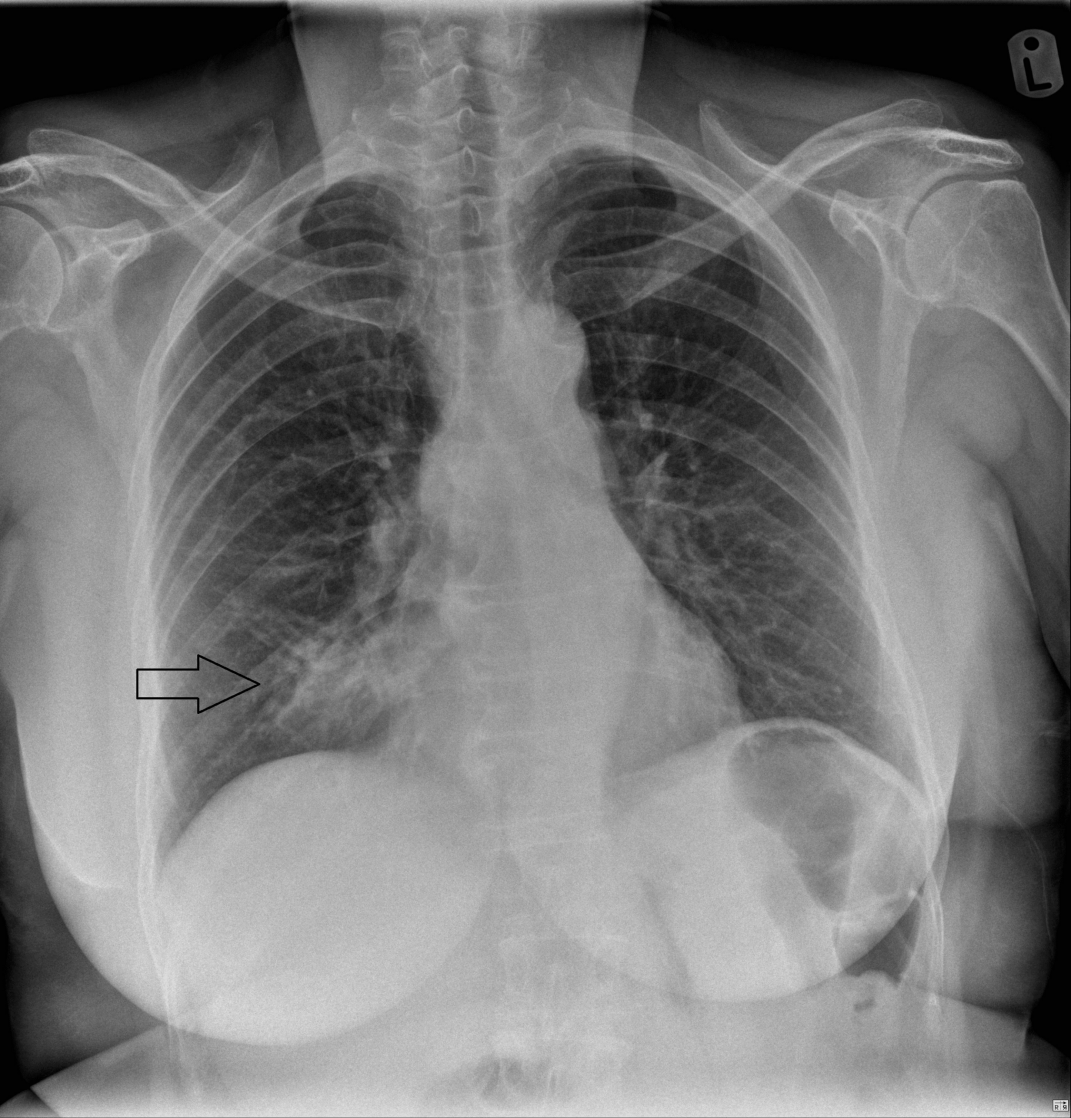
Progression of the previously demonstrated consolidation. This CXR was performed 10 days later CXR: chest x-ray
